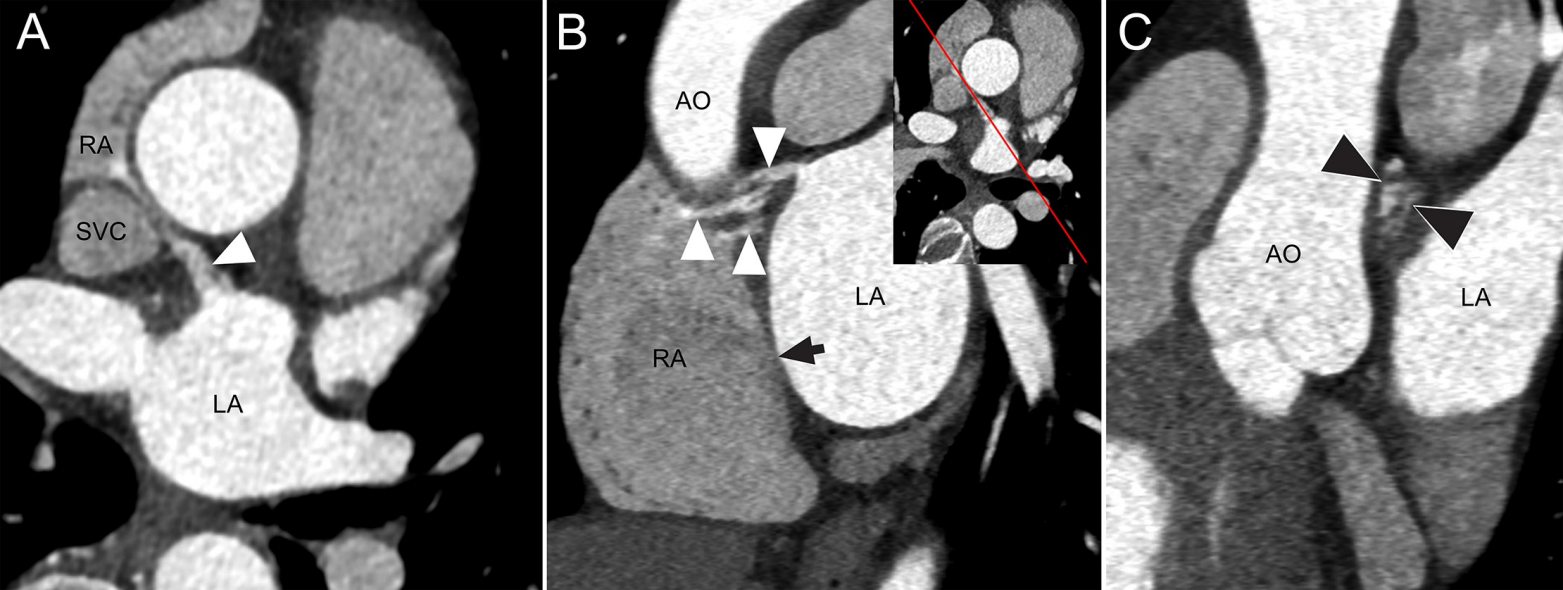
CT Images of an IAC in a 61-year-old female with Marfan syndrome. (A) Axial CT image shows a communication channel (arrowhead) between the left atrium (LA) and right atrium (RA) near the junction of the RA and superior vena cava (SVC). (B) Oblique sagittal reformatted CT image shows openings of multiple channels on the right atrial side and one channel on the left atrial side with varying thickness of the channels (2.4–4.3 mm) and length (16.5–20.8 mm). Insert image shows the direction of sagittal reformation. High attenuation of contrast-enhanced blood in the communication channels (arrowheads) indicates blood shunt from LA to RA. An arrow represents the fossa ovalis. (C) Oblique coronal reformatted image shows multiple small channels (arrowheads) in cross-section. AO, ascending aorta.
